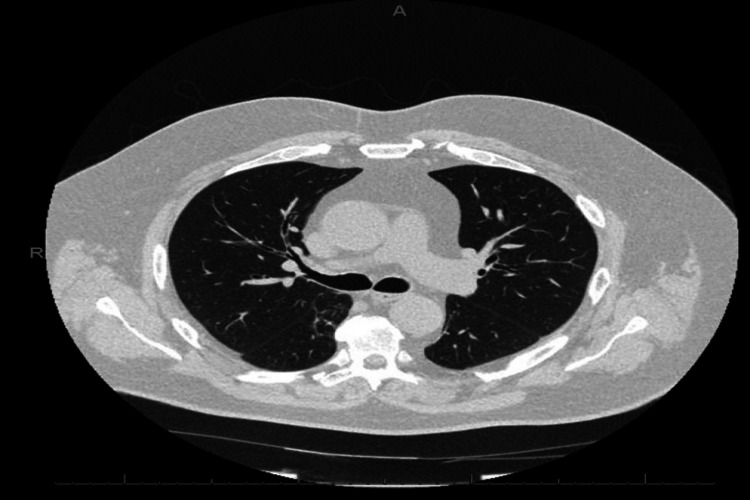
HRCT-repeat: axial view Almost complete resolution of peripheral areas of radiologically presumed organising pneumonia. HRCT: high-resolution computed tomogram
